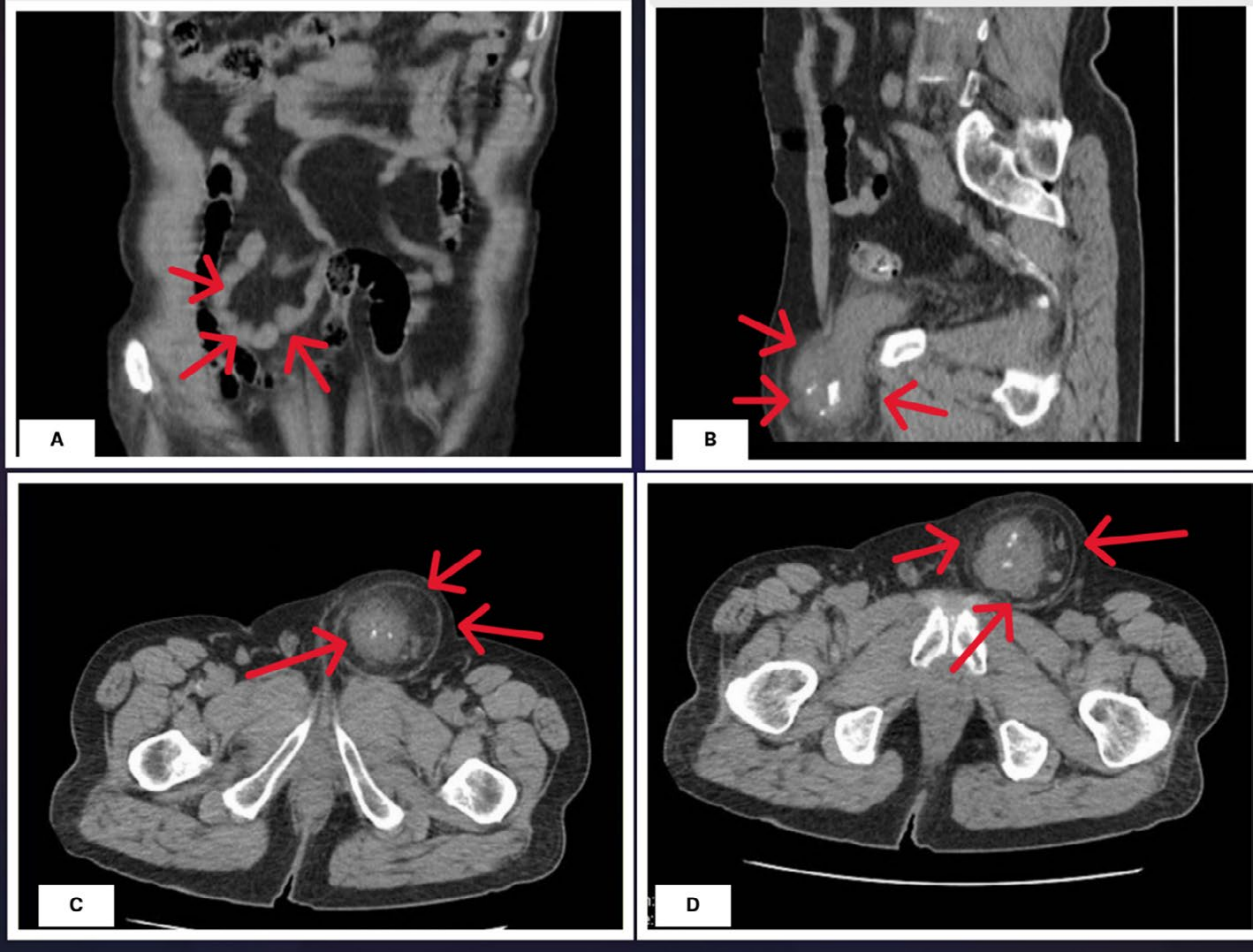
Abdominal and pelvic CT scan (with IV and oral contrast) showed a calcified, irregularly round mass (red arrows) herniating into the abdominal wall, suggesting the probability of a malignant tumor (A and B: Sagittal view, C and D: Axial view).
